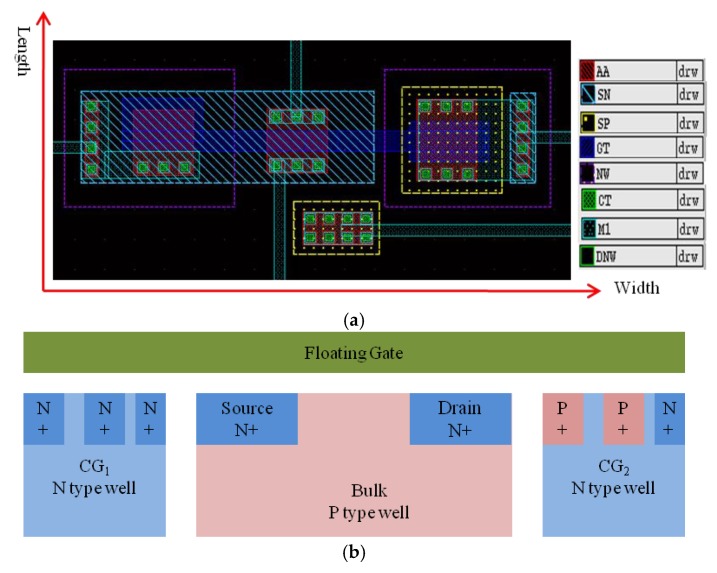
(a) Layout of the proposed ANC device, where AA is the active area, SN is the N+ implantation for the source and drain, SP is the P+ implantation, GT is the polysilicon gate, NW is the N-type well, DNW is the deep N-type well, CT is the contact area, and M1 is the metal one. (b) Cross section of the layout, where ${\mathrm{CG}}_{1}$ is a N-MOSC and ${\mathrm{CG}}_{2}$ is a P-MOSFET with a common source, drain, and substrate.
